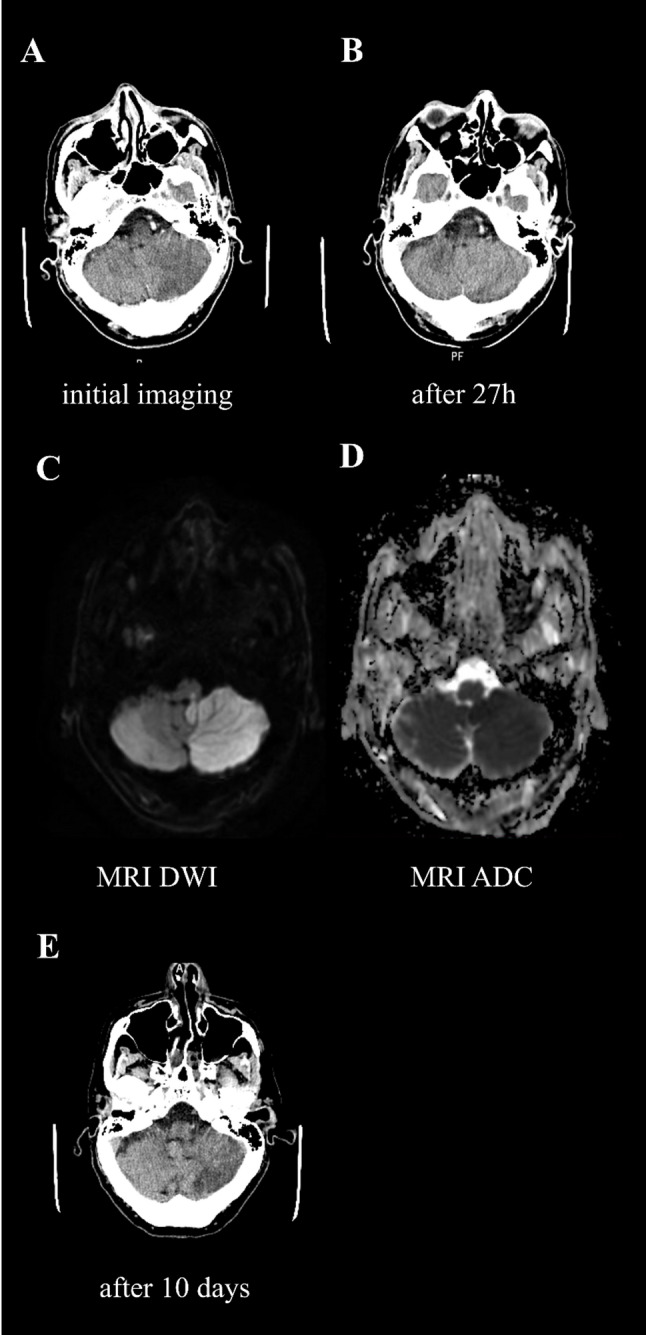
CT and MRI findings during the clinical course: Initial axial NCCT obtained at symptom onset demonstrating a left cerebellar hypodensity A; Follow-up axial NCCT performed 27 h later showing disappearance of the hypodensity B; MRI DWI (b1000) and corresponding ADC map obtained 57 h later demonstrating a left cerebellar infarction C and D; axial NCCT performed 10 days after the initial examination showing reappearance of the left cerebellar hypodensity E
